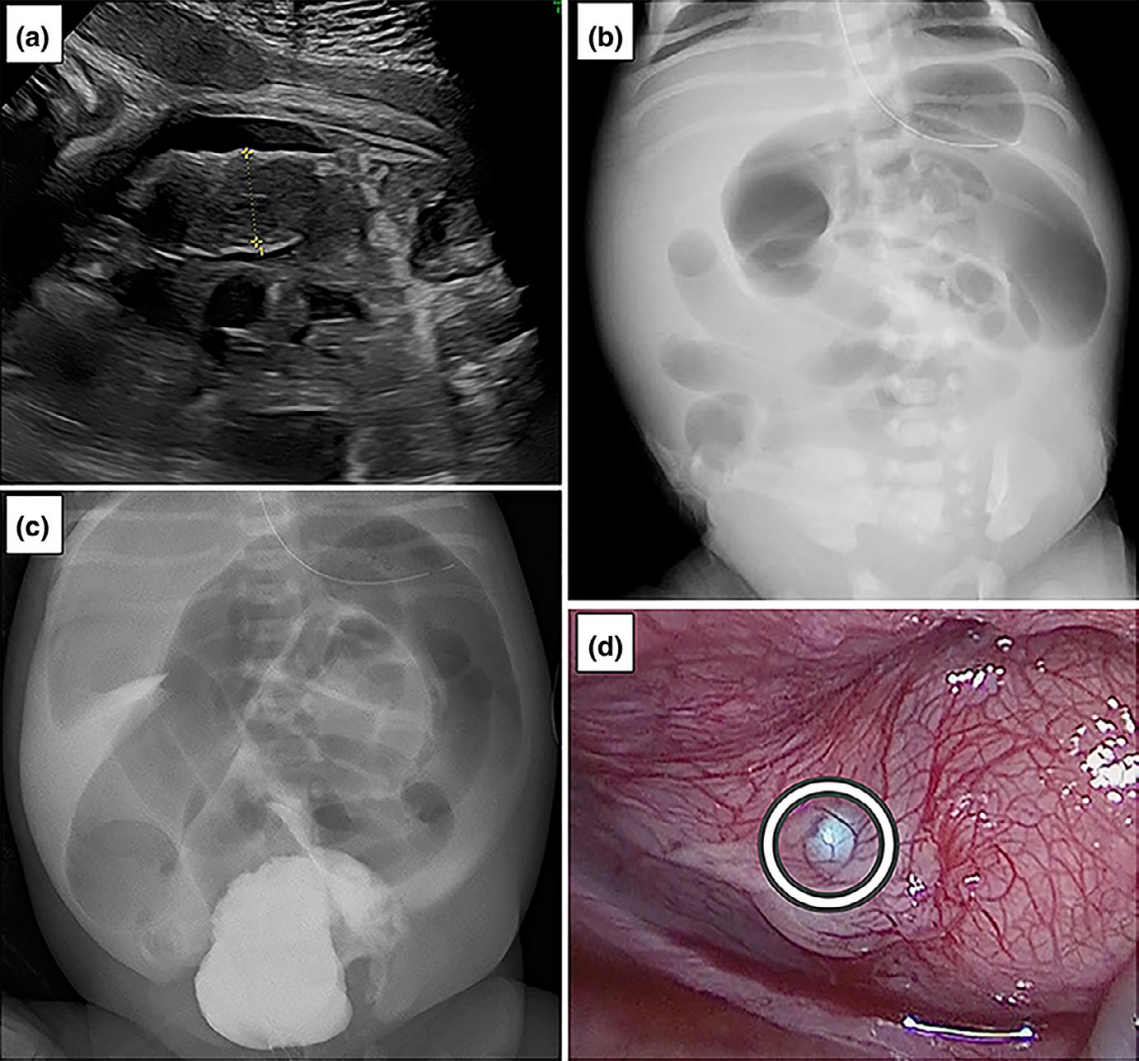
(a) Fetal ultrasound showing bowel dilation, (b) X‐ray taken after birth showing bowel dilation extending from the colon to the rectum, (c) X‐ray taken during cystography showing contrast medium leakage into the intraperitoneal space, (d) Image of the ruptured bladder.
